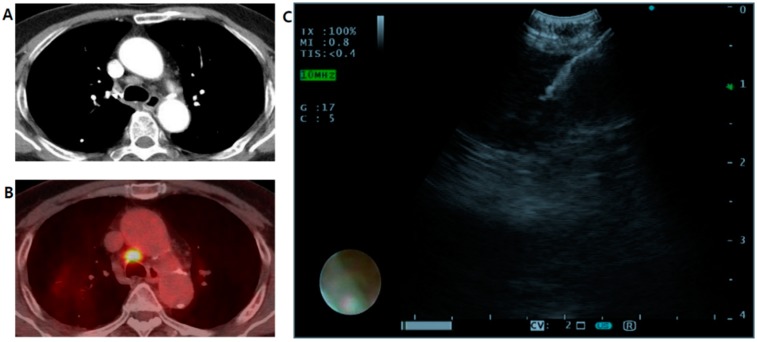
Example of a patient with a mediastinal lymph node diagnosed by EBUS-TBNA. (A,B) Enhanced chest CT and PET-CT show an enlarged hypermetabolic lymph node in the right lower paratracheal area (SUV max = 9.9). (C) Real-time TBNA of an enlarged lymph node (station 4R) under direct endobronchial ultrasonography guidance. EBUS-TBNA = endobronchial ultrasound-guided transbronchial needle aspiration; SUV max = maximum standardized uptake value.
